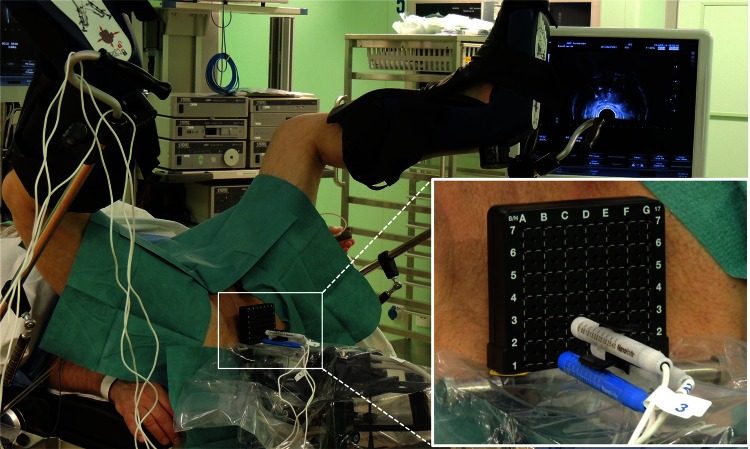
Patient in lithotomy position with three transperineally inserted electrodes under ultrasound guidance
